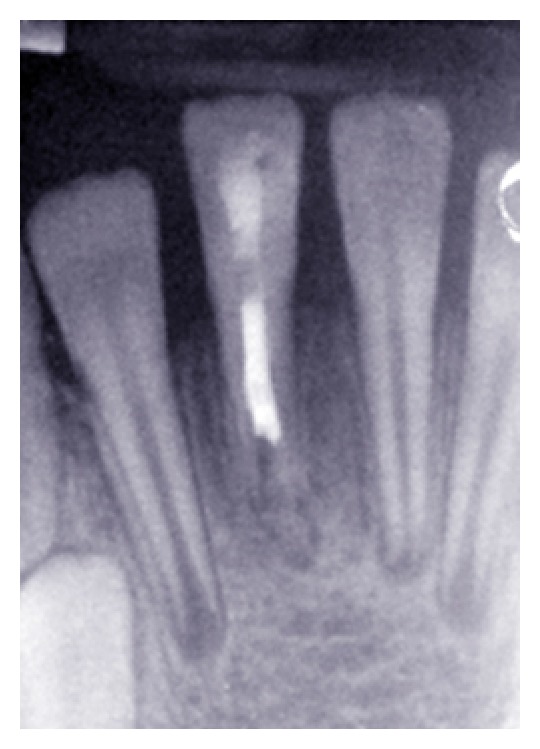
Twelve-month follow-up radiograph; continued root formation is detectable.
